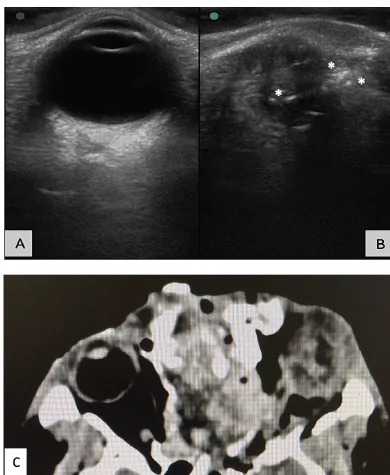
Case 3. Ocular ultrasound modified water bath technique performed: A) right normal eye; B) left globe rupture with intraocular foreign body seen as echogenic structures with posterior acoustic shadowing (marked with asterisks); and C) computed tomography suggestive of left globe rupture.
